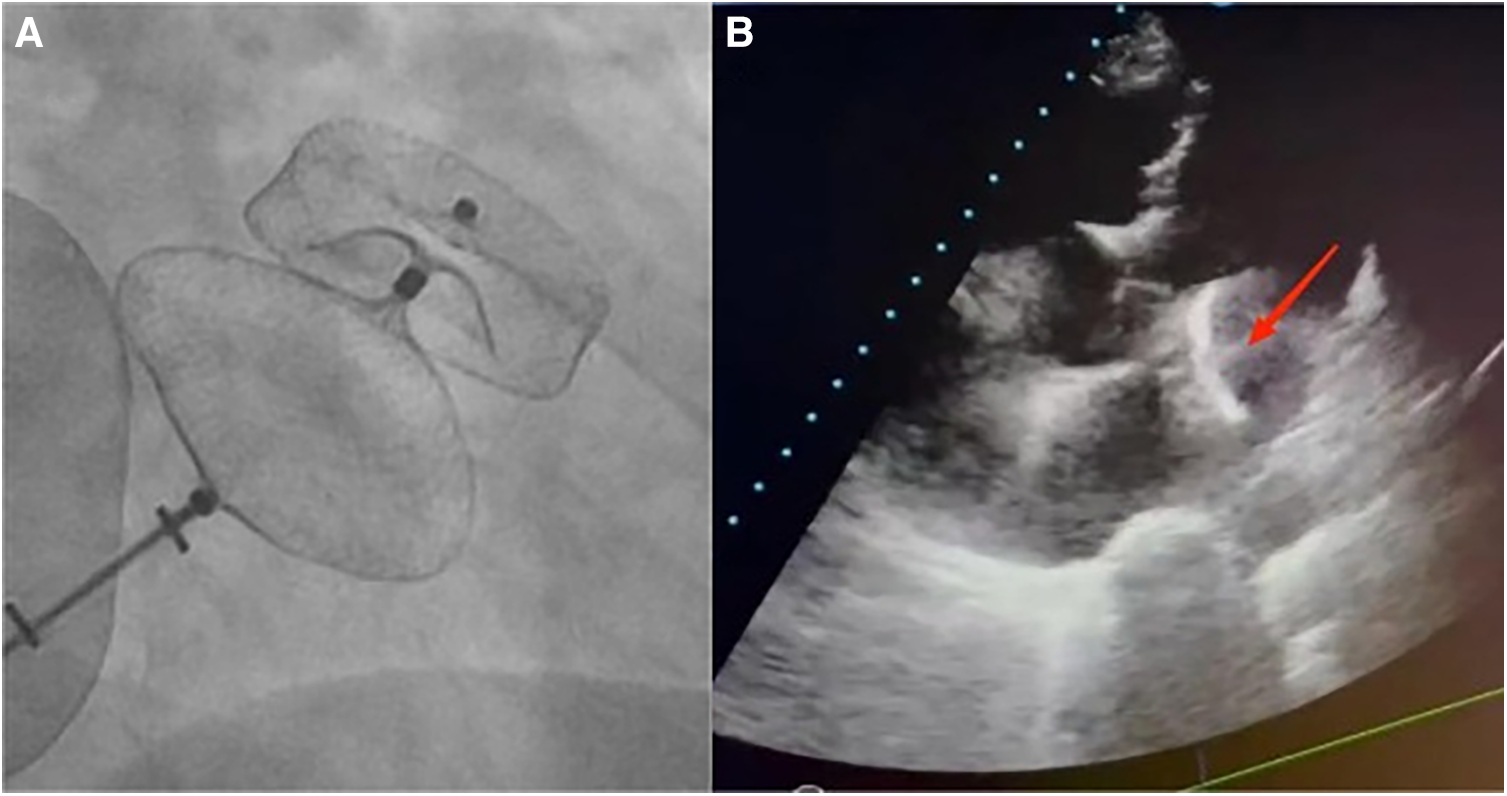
(A) A fluoroscopic image of a 34 mm LACbes device deployment in the LAA shows that the device is well-positioned with no significant residual shunting. Postoperative angiography confirmed this, and the tug test indicated good stability of the LACbes device. (B) Echocardiography reveals a migrated LAAO within the LA, with an arrow indicating its floating position.
